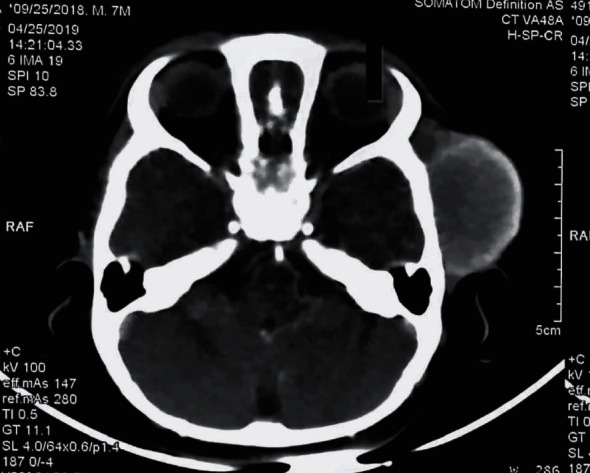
CT scan brain showing soft tissue mass in right temporal area under the skin not involving bone and brain.
